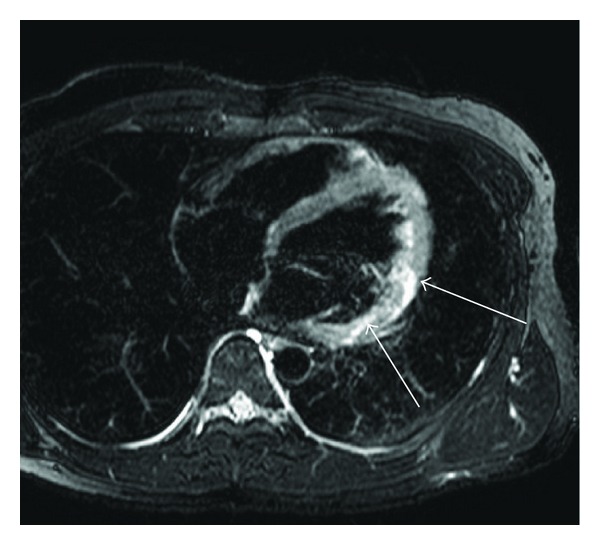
25-year-old male with acute myocarditis. T2-weighted MRI shows myocardial edema associated with acute myocarditis (arrows). The edema predominantly involves the epicardial or transmural myocardium in the lateral wall.
